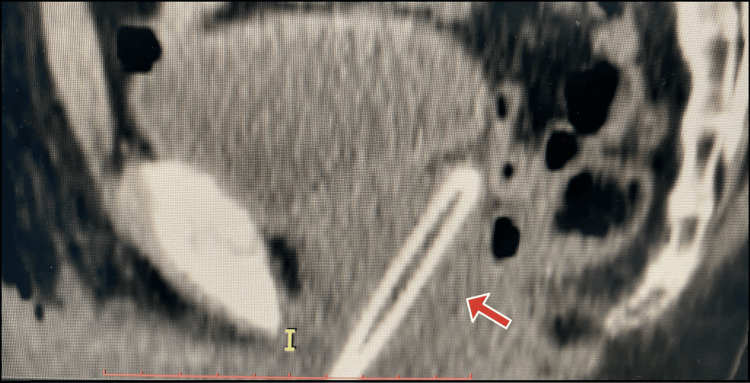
CT image showing the penetration of the prostatic gland by the inserted object CT: computed tomography
